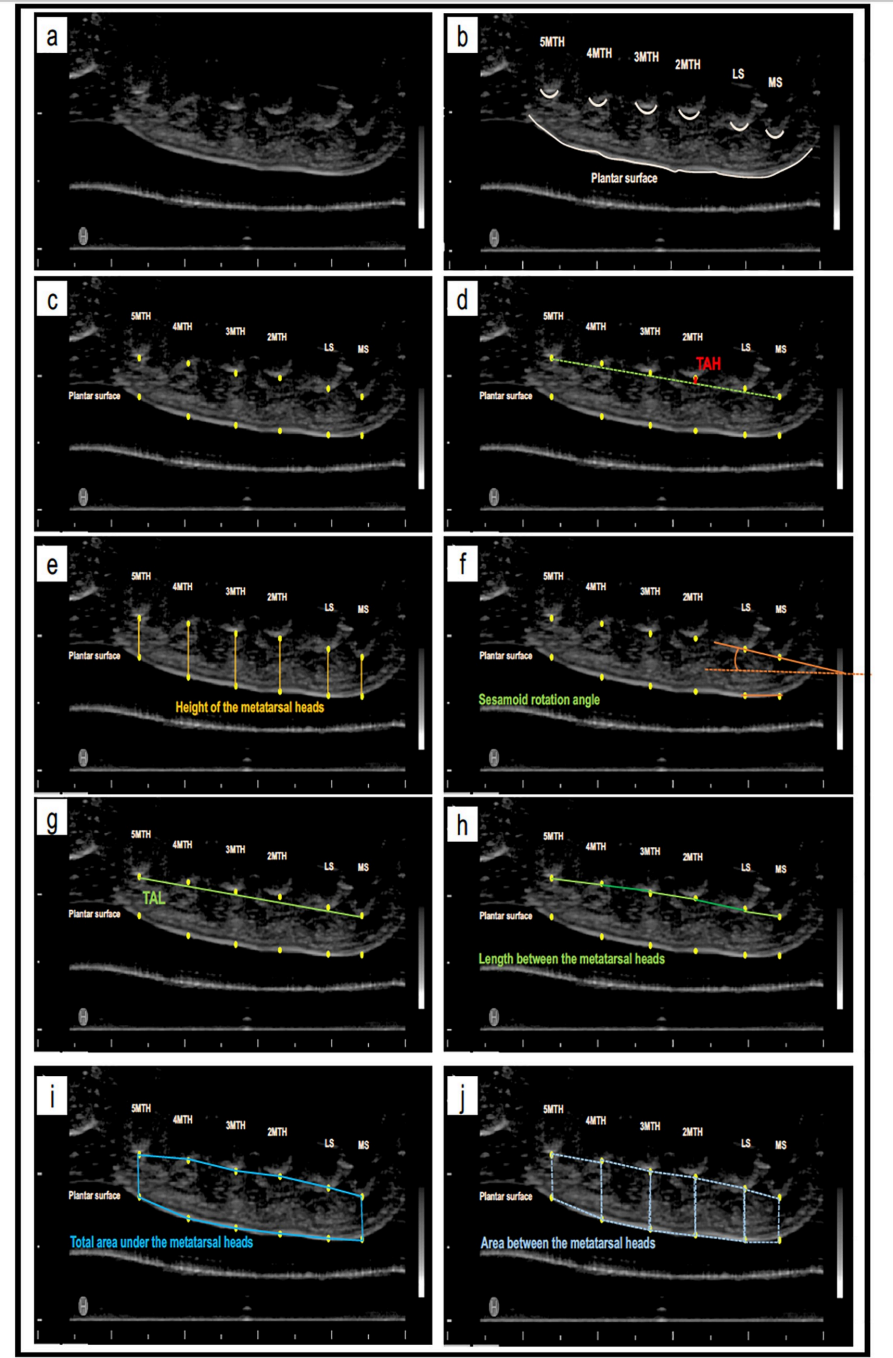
Ultrasound image analysis. a) ultrasound image of the transverse arch; c) marking the lowest points of MS, LS, 2MTH, 3MTH, 4MTH, 5MTH and their plantar surface projections; d) TAH is red line; e) the height of the metatarsal heads in orange lines; f) SRA in orange angle; g) TAL in green line; h) the length between each metatarsal head in green lines; i) the total area under the metatarsal heads marked by blue lines; j) the area between each two metatarsal heads marked by dotted blue lines.
